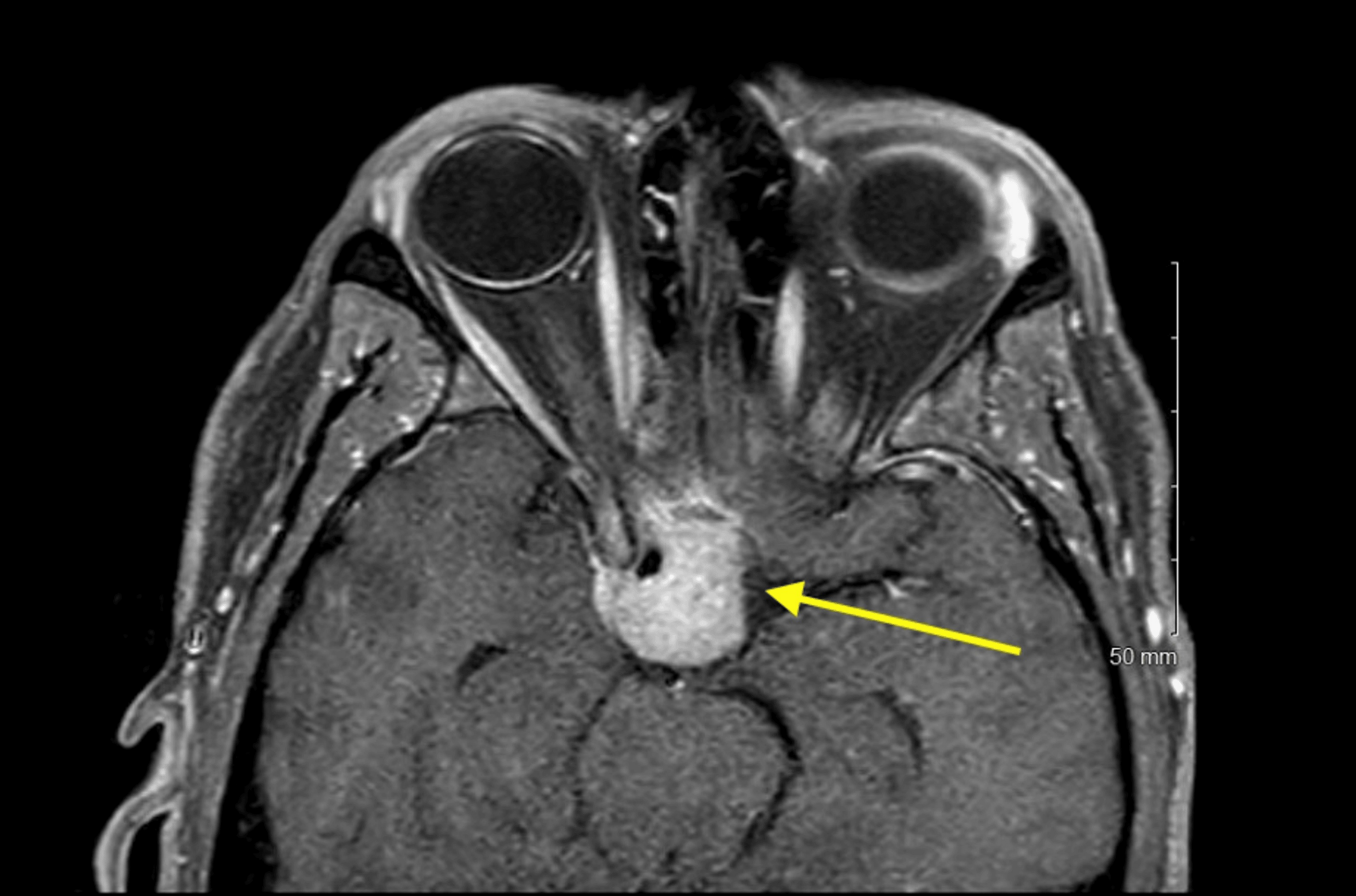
Axial T1 MRI of the orbits with intravenous gadolinium contrast and fat suppression series showing slight extension of tumor into the right cavernous sinus and tumor contacting the prechiasmatic optic nerves
